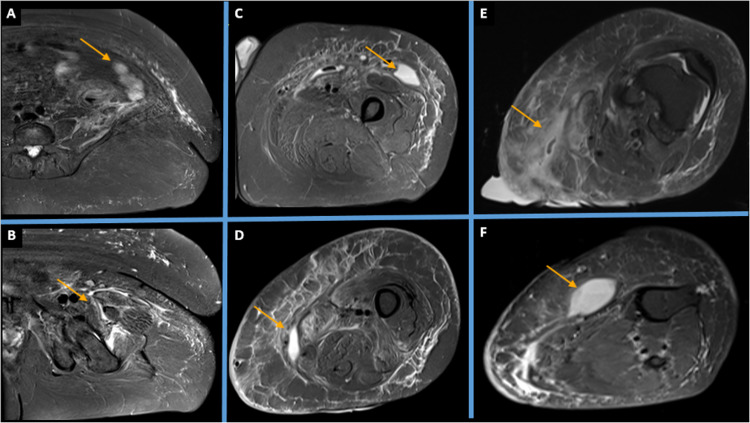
Day 22 MRI Axial short tau inversion recovery (STIR) images at the level of (A) pelvis, (B) proximal left thigh, (C) mid left thigh, (D) lower left thigh, (E) left knee joint, and (F) proximal left leg, with orange arrows showing infective fluid pockets.
